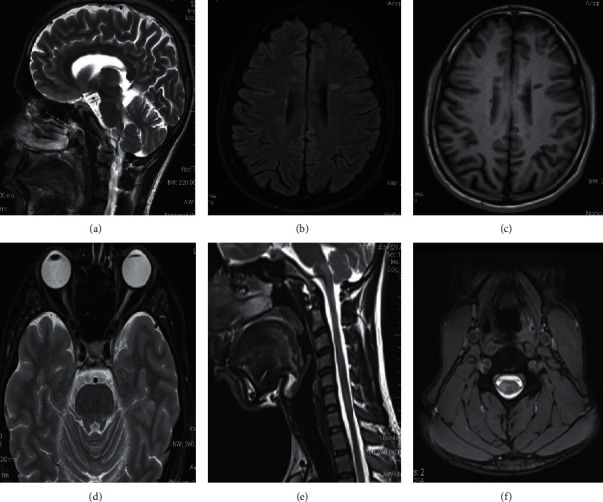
MRI brain and spine of son: (a) T2 sagittal, (b) T2 FLAIR axial, (c) T1 axial images demonstrate numerous T2 high signal T1 low signal white matter lesions arranged perpendicular to the bilateral lateral ventricles suggesting Dawson's fingers. Lesions involve the corpus callosum, callasoseptal interface, and posterior fossa. T2 axial image of the orbits (d) shows right optic neuritis. Cervical spine T2 sagittal (e) and axial (f) images demonstrate multiple short segment T2 high signal lesions without significant spinal cord edema and expansion. Lesions are not contrast enhancing.
